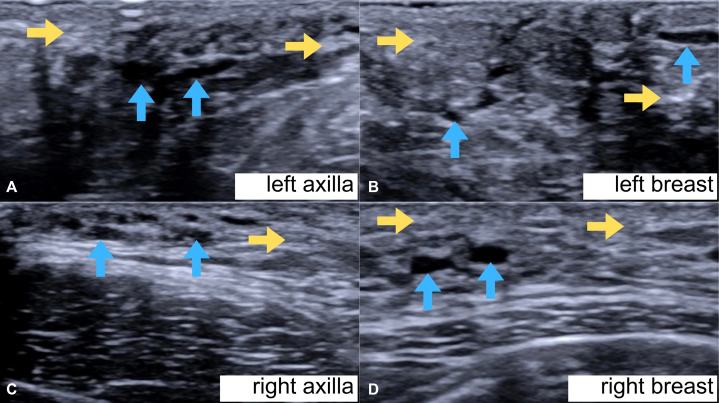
Focused ultrasound of bilateral axillae (A, C) and breasts (B, D). *Yellow arrows* point to fibroglandular parenchyma while *blue arrows* show dilated ducts.
